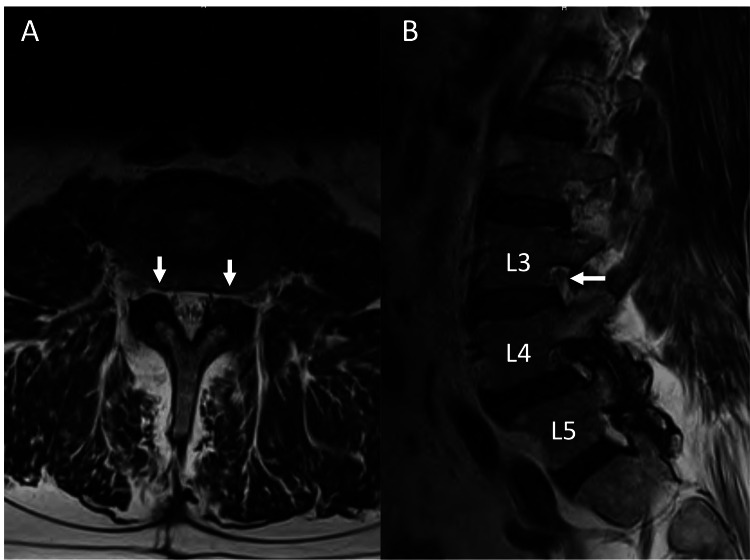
Pre-operation MRI. A: Axial view demonstrated bilateral neuroforaminal stenosis at the L3/4 level (arrows). The radicular symptom was more severe on the left side and we performed left full-endoscopic transforminal decompression. B: Sagittal view revealed that the left L3 exiting nerve root appears to be high in the L3/4 foramen (arrow). During the surgery, the L3 exiting root turned out to be low. This emphasizes that we could not purely rely on MRI to determine the position of the nerve root.
